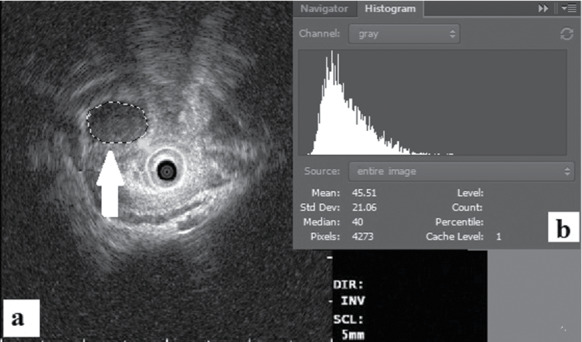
Endoscopic ultrasound combined with grayscale histogram (a, b).
